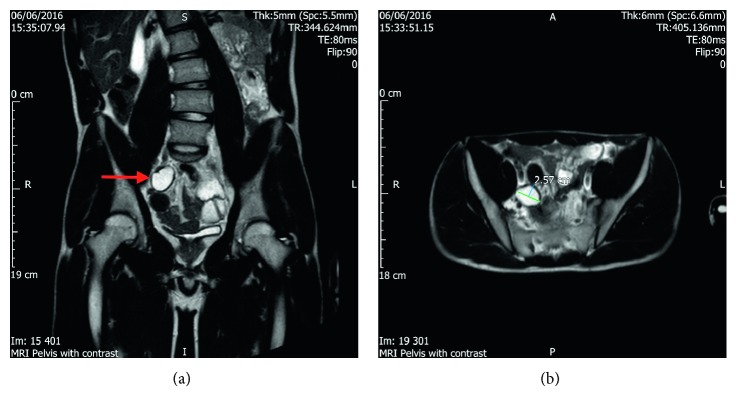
Subsequent MRI (with contrast) images.
